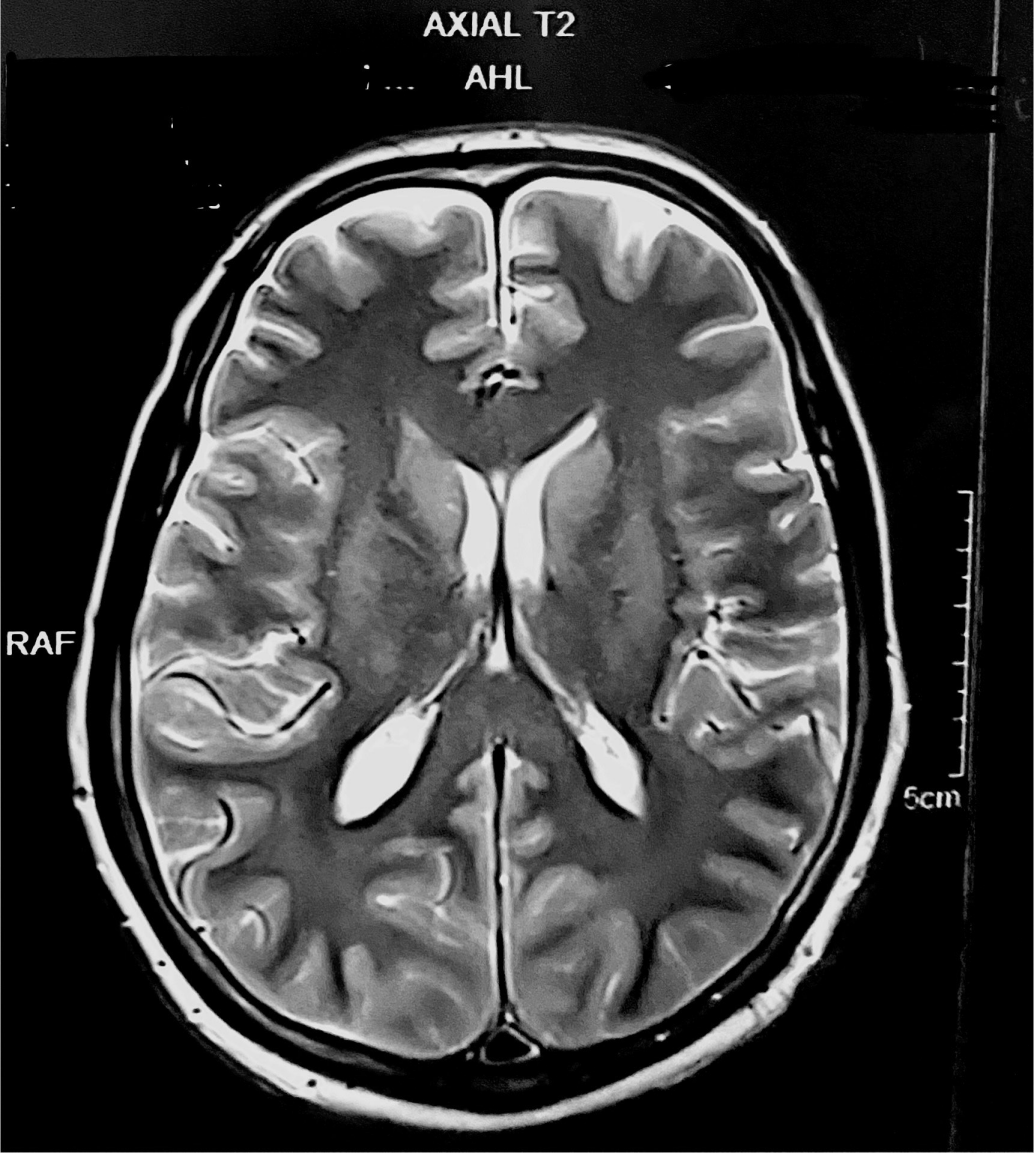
Axial T1 view of MRI brain.
